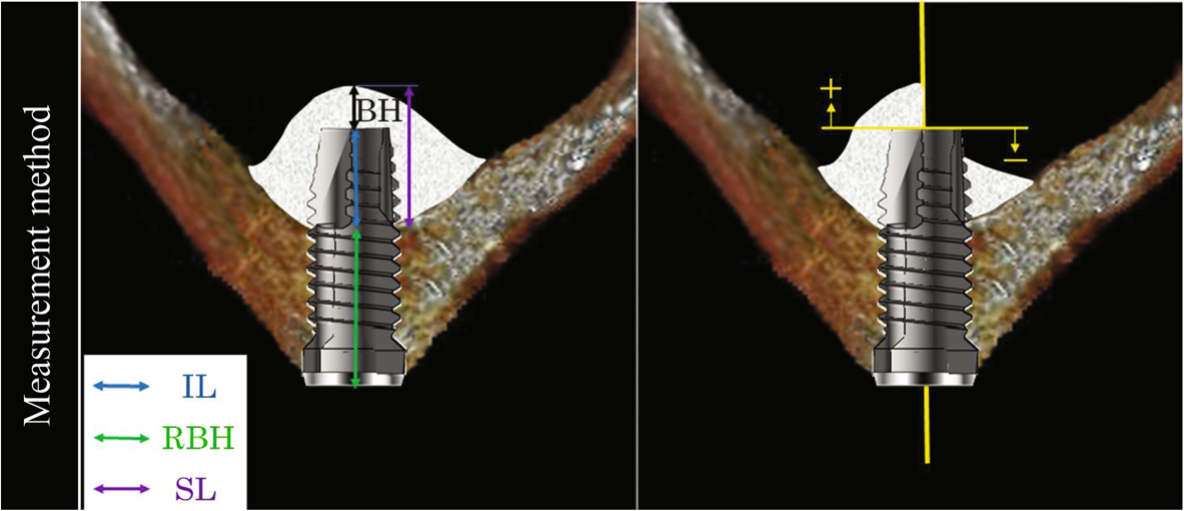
Measurement method using CBCT. Radiographic examination on the coronal cross-section images parallel to the longitudinal direction of the implant. The linear variables include height of the augmented bone above apex of the implant (BH), height of the augmented sinus floor (SL), implant length that projected into the sinus (IL), residual bone height (RBH)
